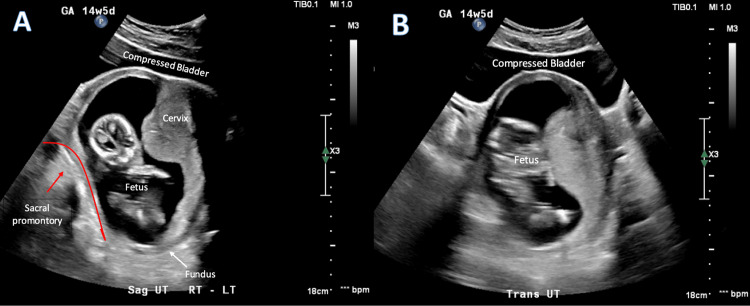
Comprehensive transabdominal ultrasound images of incarcerated gravid uterus in the sagittal and transverse plane A) Sagittal image demonstrates the superiorly displaced cervix and lower uterine segment above the uterine fundus, with superior displacement of the urinary bladder and resultant bladder outlet obstruction. The fundus is trapped below the sacral promontory (marked by the red line). B) Transverse image redemonstrates bladder outlet obstruction due to the upward compression of the displaced uterus on the urinary bladder.
